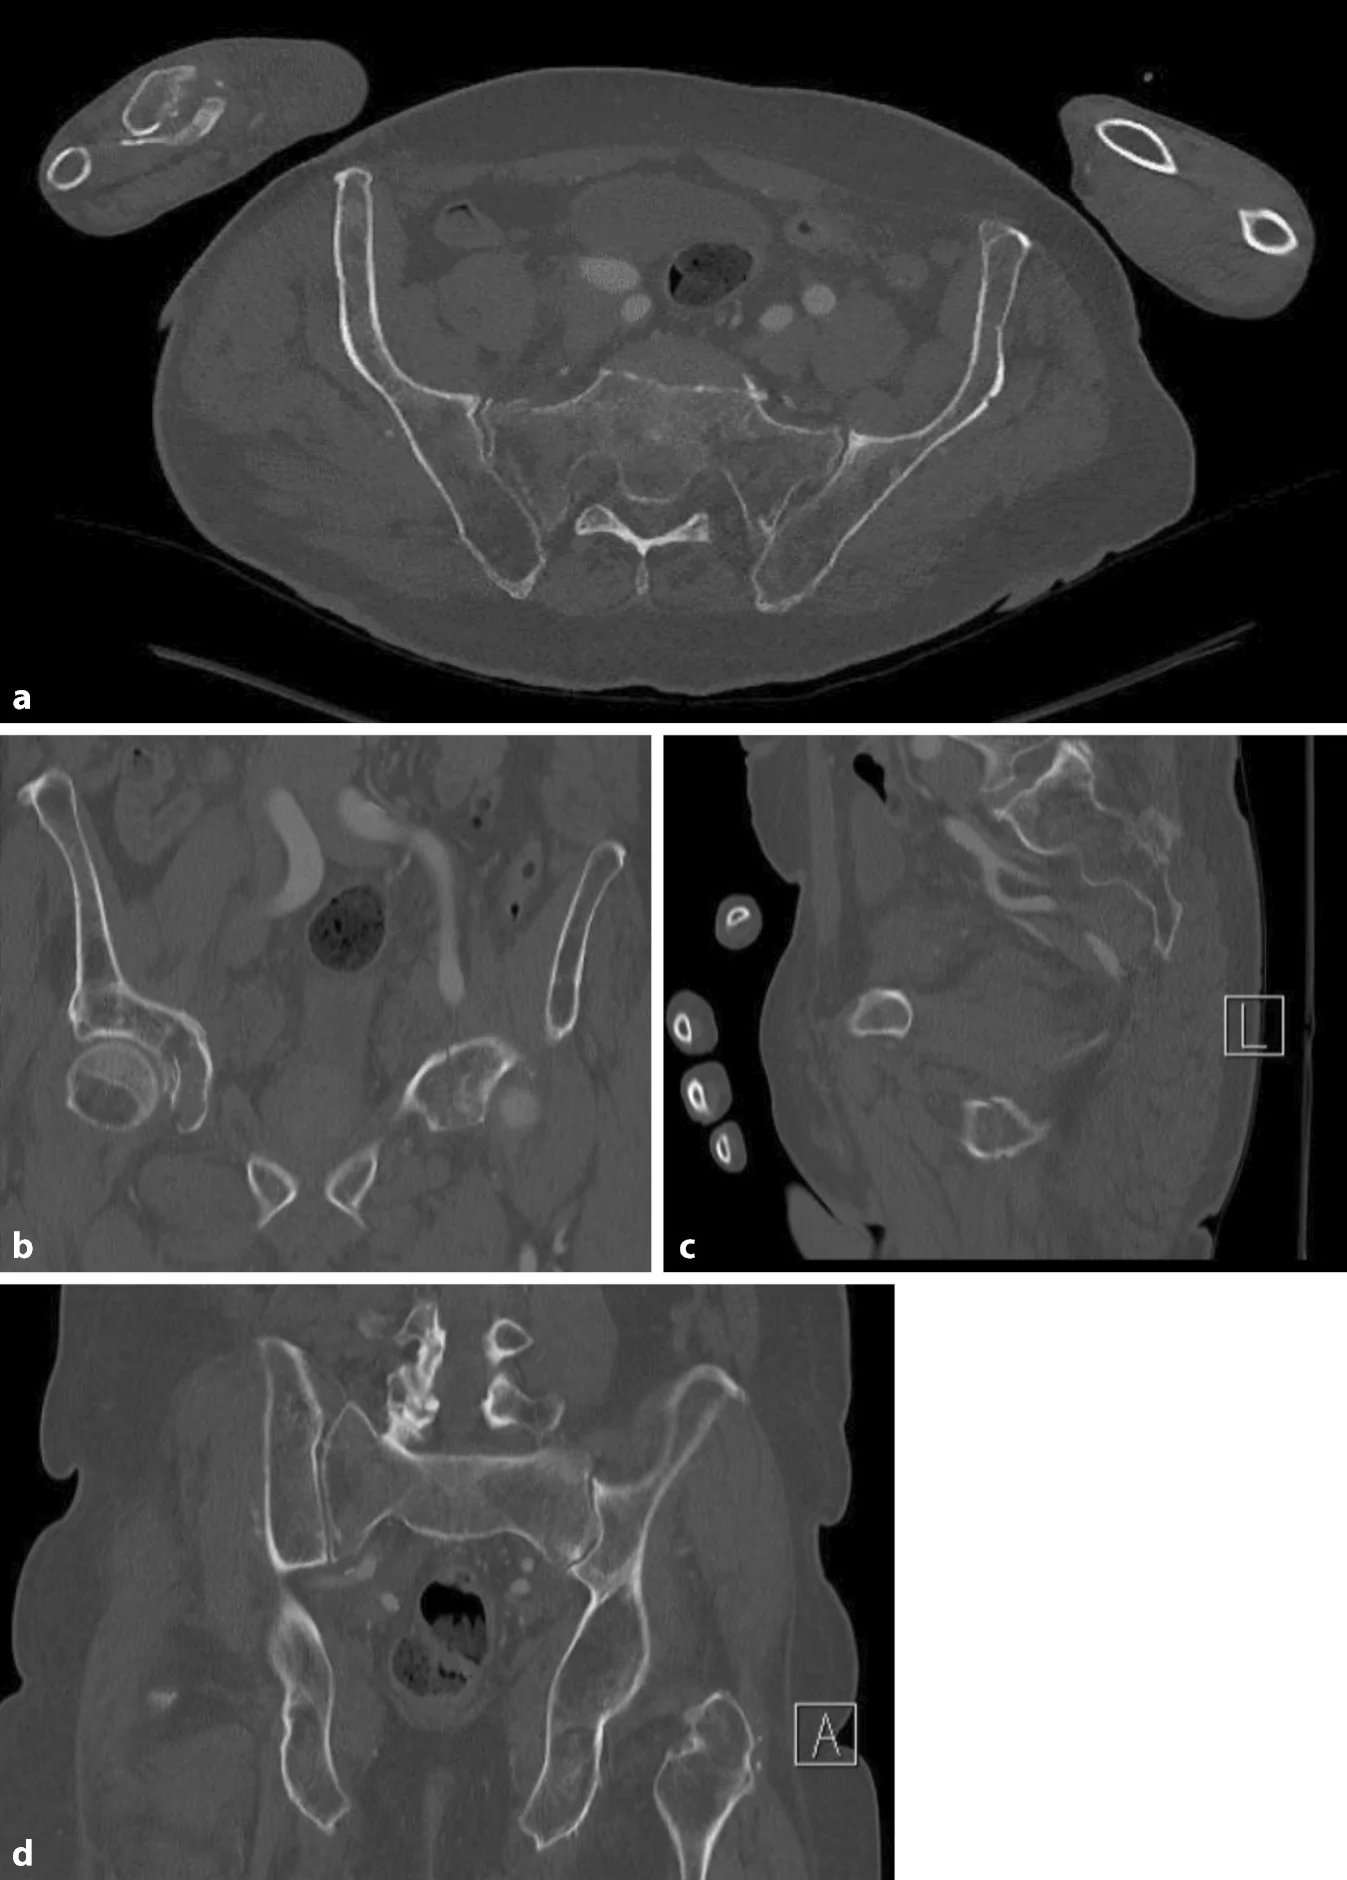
Präoperatives CT der instabilen Beckenringfraktur mit beidseitigen Frakturen des oberen und unteren Schambeinastes sowie H‑förmiger Sakrumfraktur (a axial, b und d koronar, c sagittal)
